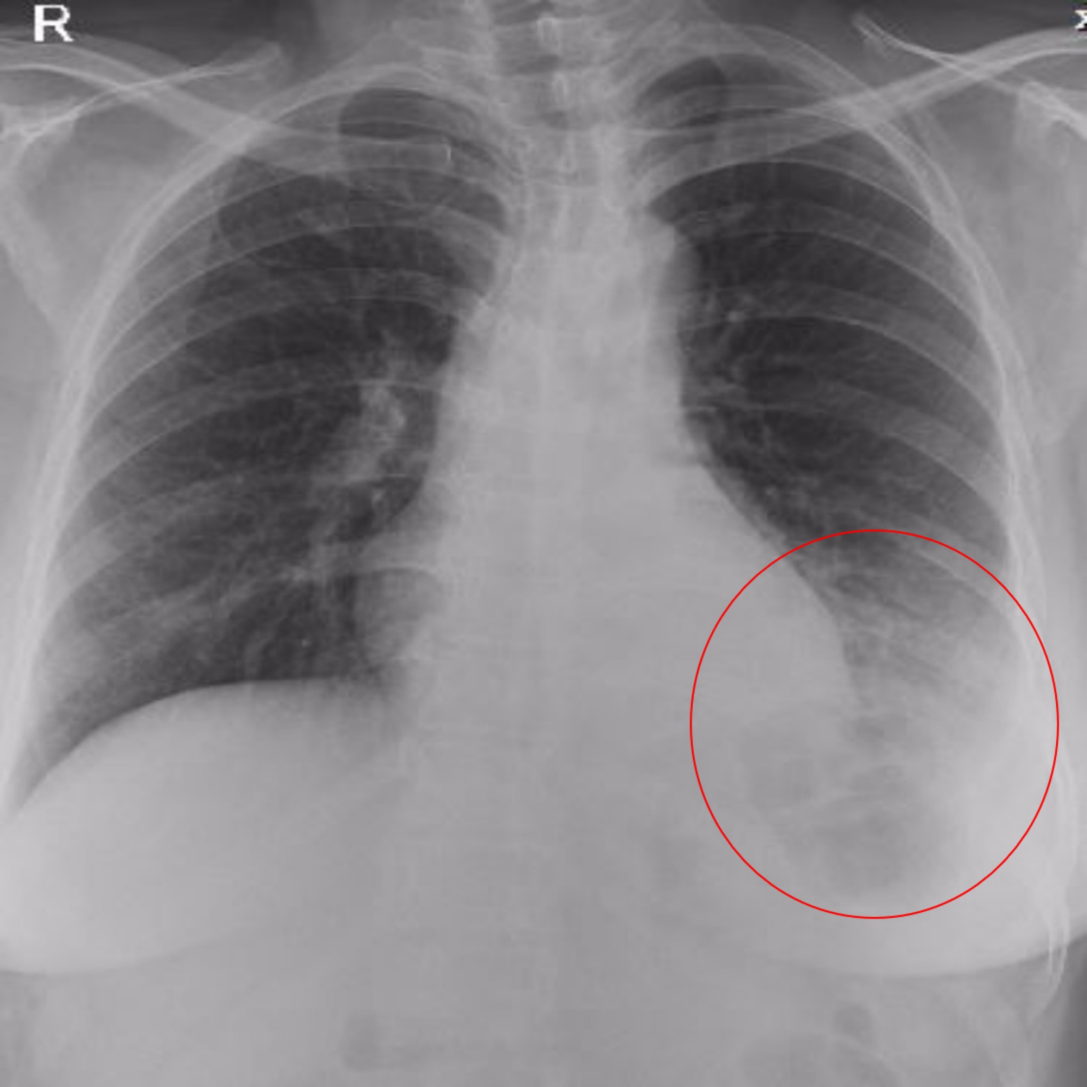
Chest roentgenogram showing diffuse opacity in the area of the left lower lobe
